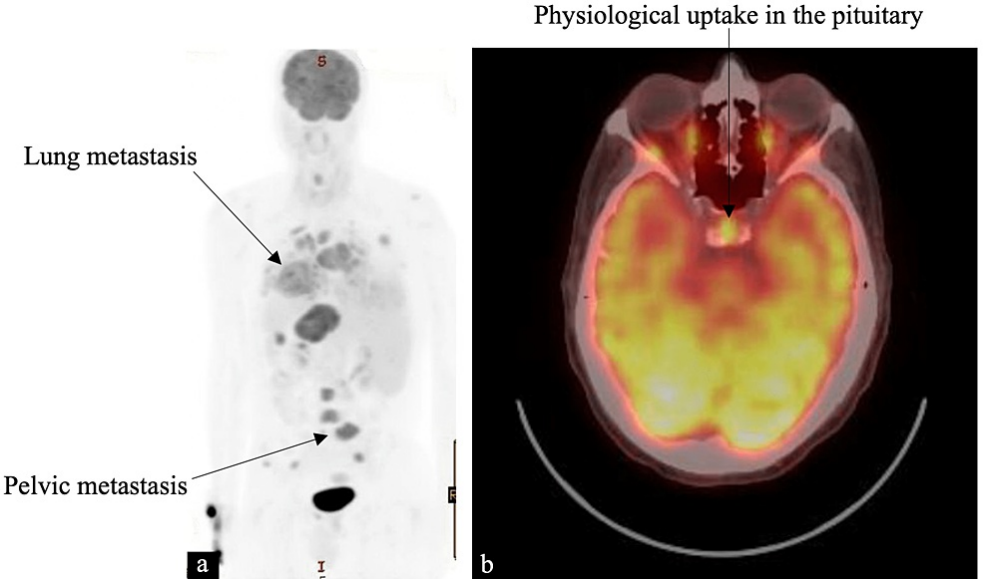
FDG-PET scan (a) Diffuse FDG avid disease in the bones and lungs. (b) Physiological FDG uptake in the pituitary gland (arrow). FDG, fluorodeoxyglucose; PET, positron emission tomography
